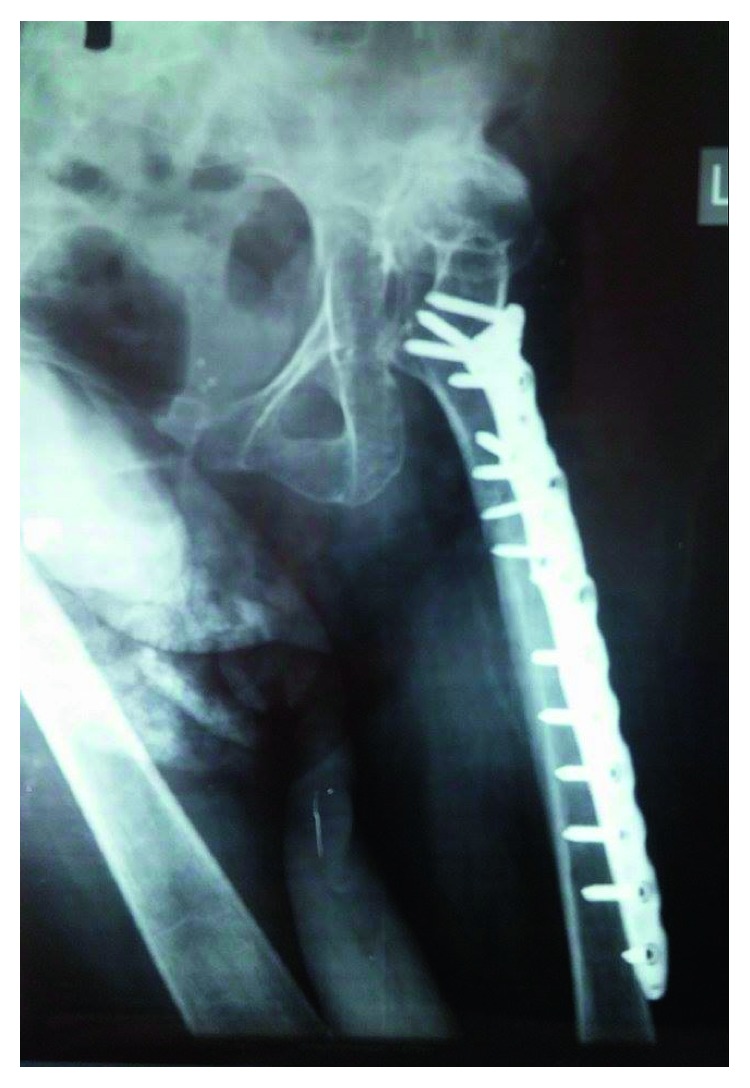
Profile radiograph of the left femur six weeks postoperatively.
